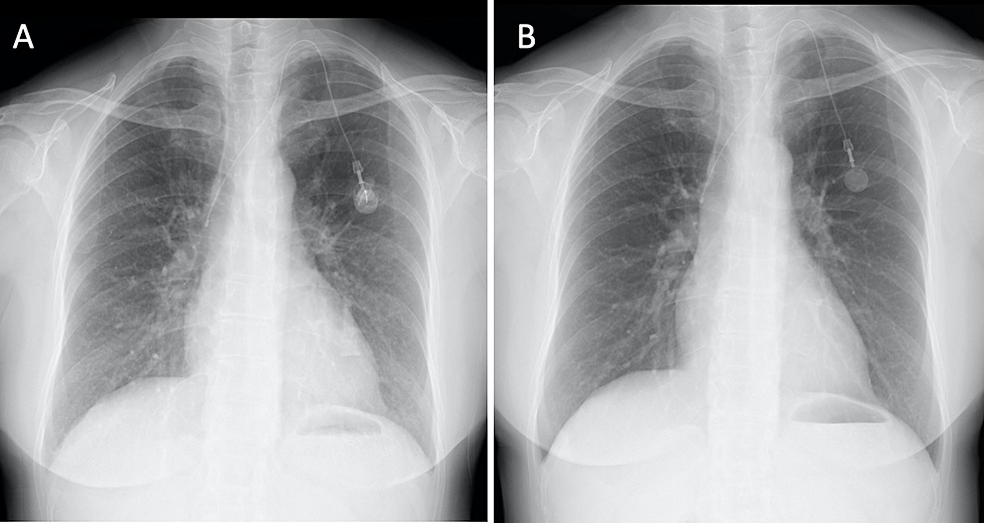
Chest radiographic images of the patient. Chest X-ray images (A) on admission and (B) at discharge.
